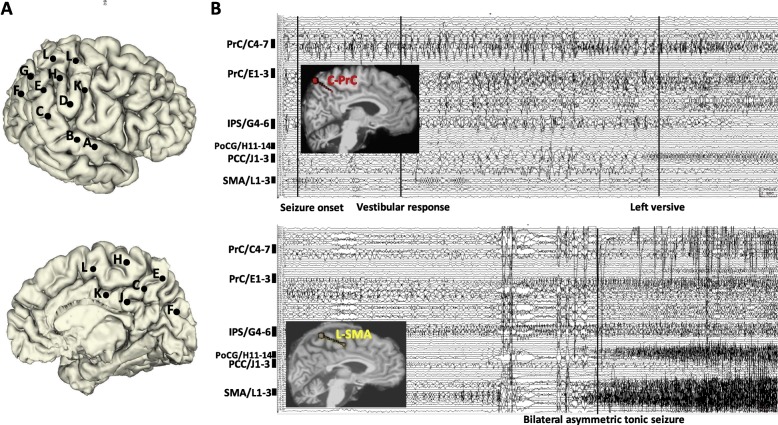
a SEEG electrode implantation on lateral and medial view of 3D brain of Patient 3. b Ictal SEEG trace and 2D T1-weighted sagittal images with 3D electrodes showing the key target points. It shows that the seizure onset was recorded from a relatively focal region of PrC (middle contacts of electrode C4–7 and mesial contacts of electrode E1–3). It is only when the seizure spreads to PoCG (lateral contacts of electrode H11–14) and SMA (medial contacts of electrode L1–3), that the semiology of BATS appears
